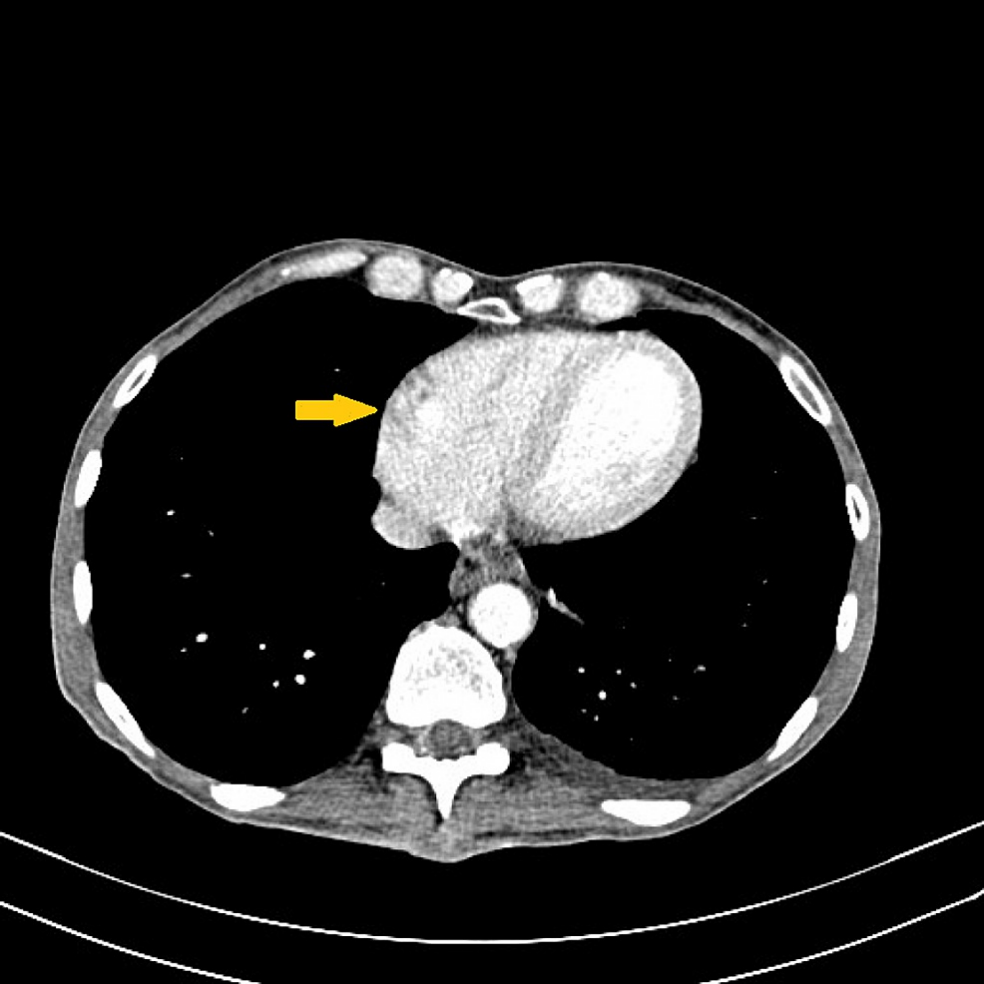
CT scan image showing the partial absence of the pericardium covering the right side of the heart (yellow arrow).
